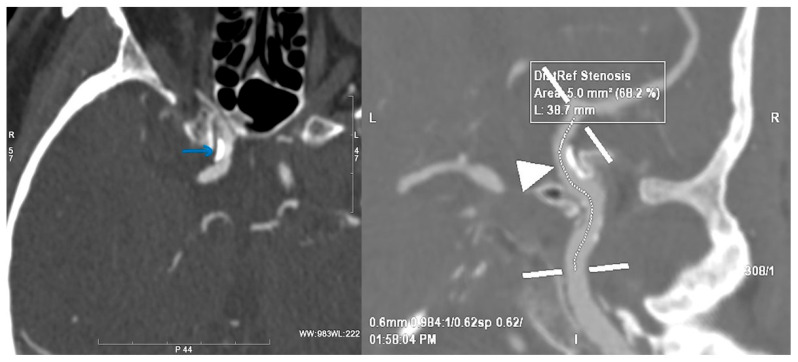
The ratio on CT angiography—62.8% (“R” for right side of the image, “L” for left side of the image).
